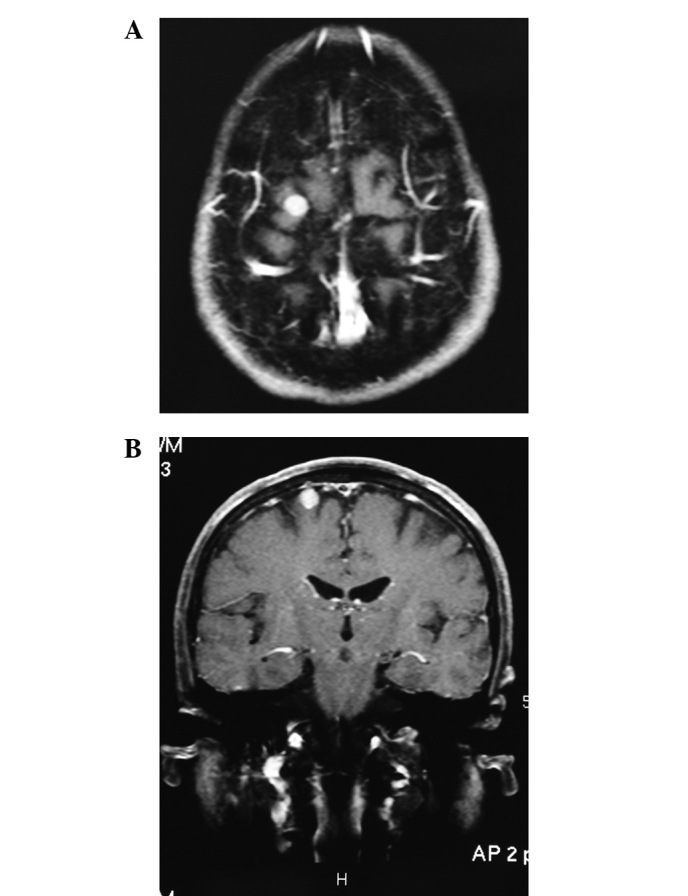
(A and B) Case 1: A metastatic lesion was identified in the right temporal lobe of the cerebral hemisphere during a follow-up magnetic resonance imaging (MRI) examination. (A) Transverse section; (B) coronal section.
